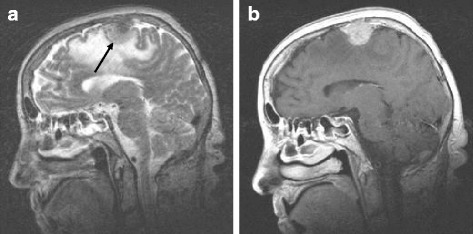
A sagittal T2-weighted spin echo image showing a cortical meningioma in the motor area (*arrow*) surrounded by extensive oedema (a). On T1-weighted spin echo image with contrast application (b), the tumour enhanced homogenously
